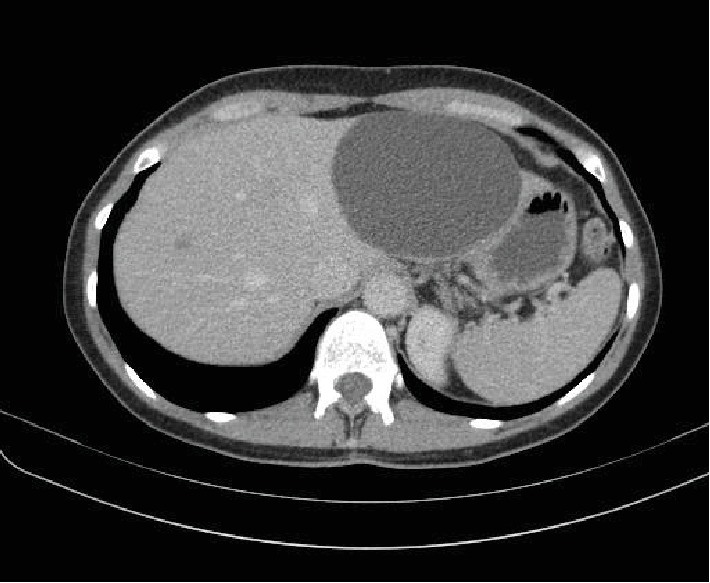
CT scan: large hepatic cyst in the left hepatic lobe.
